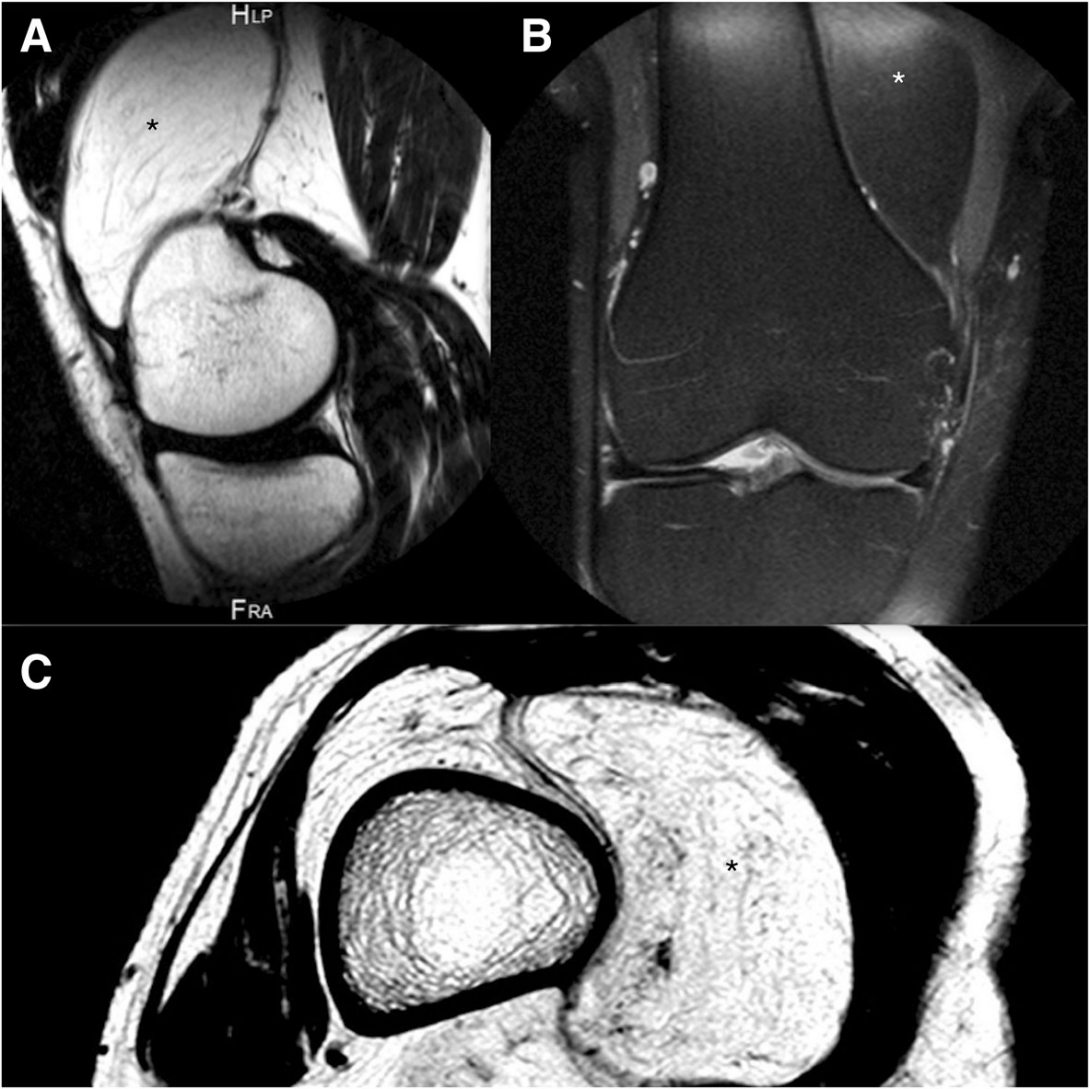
MRI of the knee shows an intraarticular lipoma (*): a): T1-w image in Sagital view. b: T2-w FS image in Coronal view. c T1-w image in Axial view at the level of the suprapatellar pouch
